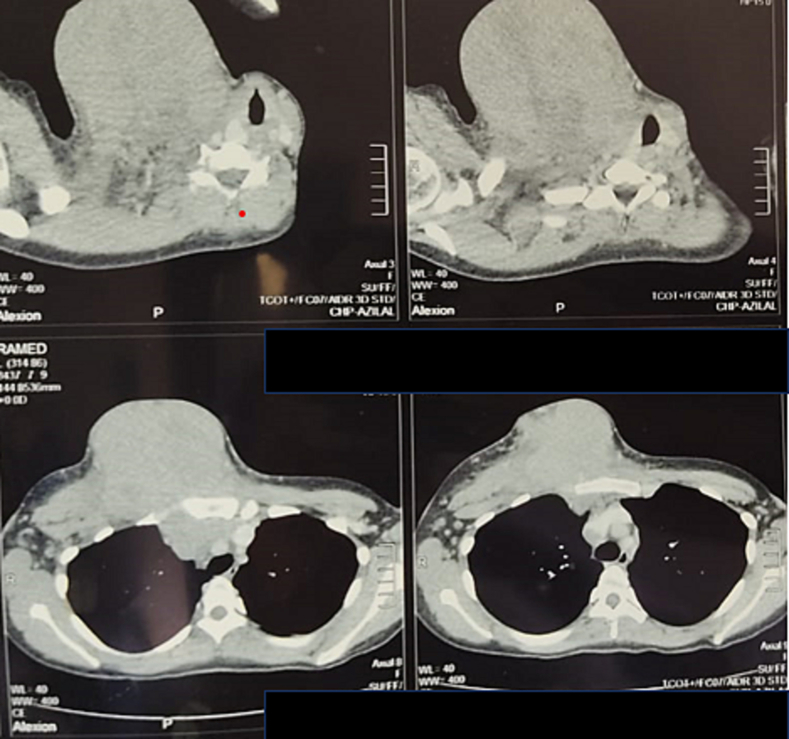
Chest CT scan before chemotherapy showing a large tissular tumor of 15 cm along its long axis in front of the right medial clavicle.
